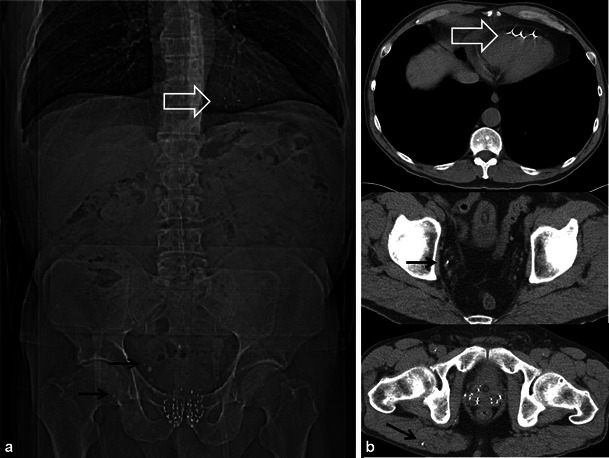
A 64-year-old man with prostate carcinoma treated with brachytherapy. Routine follow-up unenhanced CT reveals multiple radio-dense seeds in the prostate and several which have migrated out of the prostate revealed both on the scout tomogram (a) and axial CT images (b). Seeds are located in the right gluteus maximus and right pelvic veins (*black arrows*) and embedded in the right ventricle (*open white arrows*). Seed migration is common, occurring in up to 25 % of patients and should be noted on follow-up imaging
